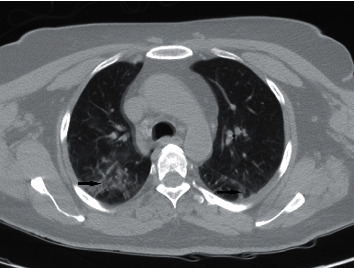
Noncontrast thoracic CT scan (axial view) showing bilateral lung infiltrations (black arrows).
